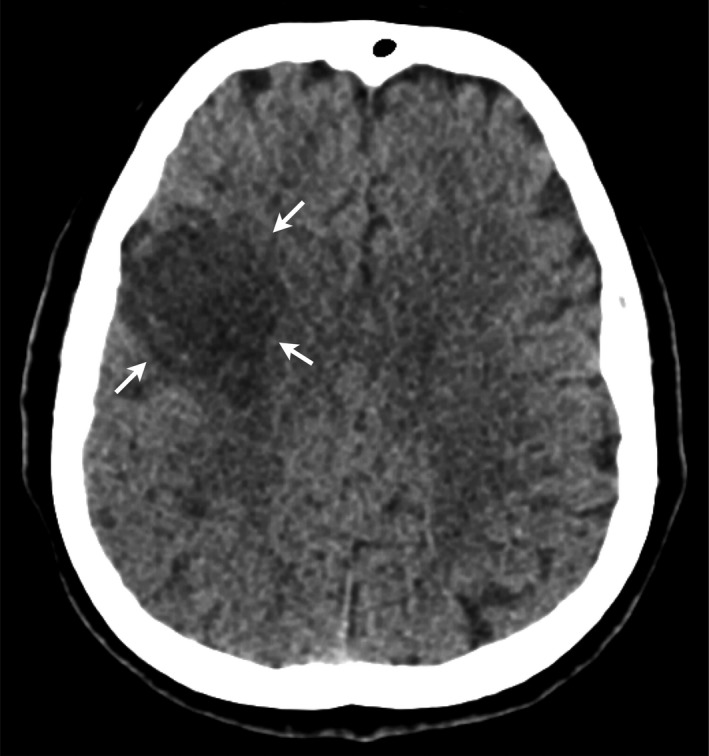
Axial noncontrasted computed tomography demonstrates a diffuse hypodense area in the inferior frontal and precentral gyrus in the middle cerebral artery territory.
